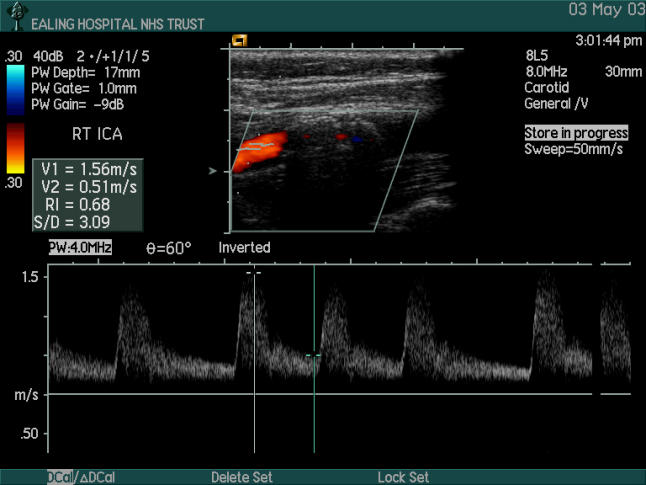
Carotid Artery Ultrasound Showing a Completely Calcified Atherosclerotic Plaque (Geroulakos Type 5) Although post-stenotic velocities are increased, accurate grading with ultrasound is not possible.
